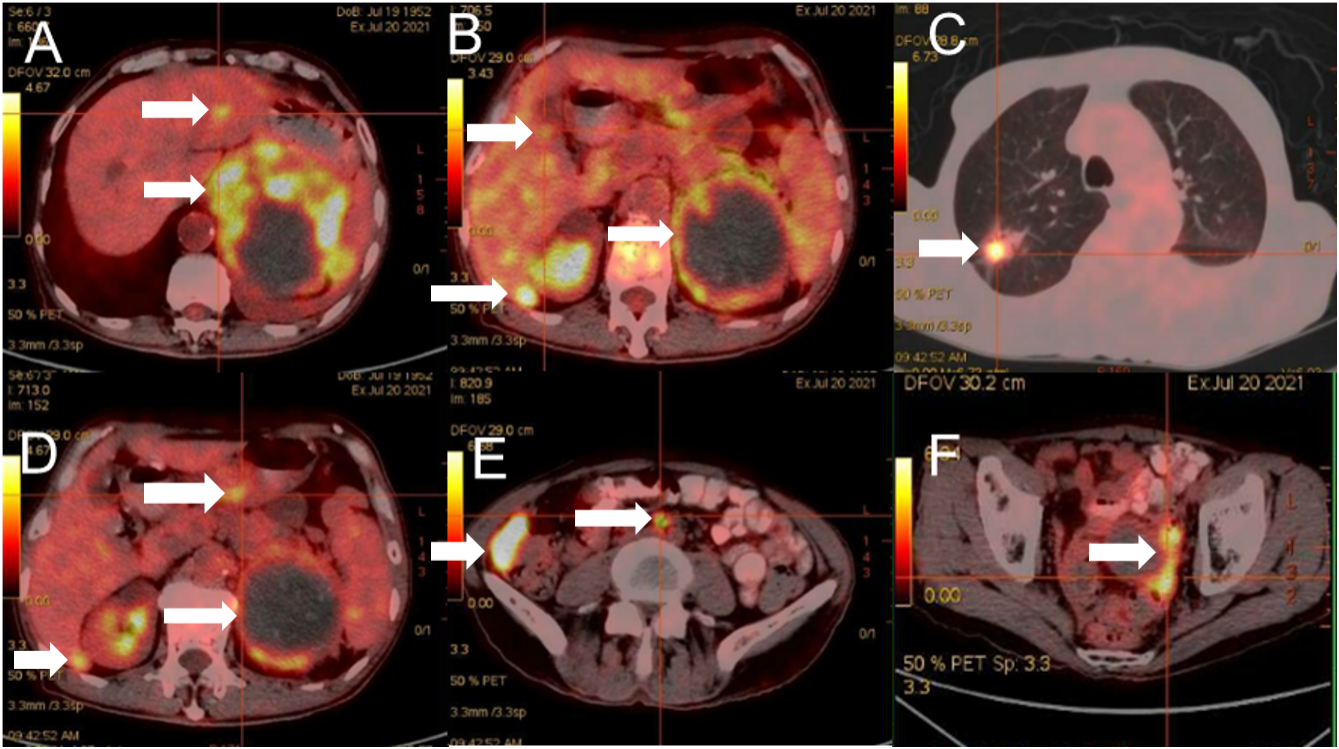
Positron emission tomography-CT showed a large, multi-loculated, hypermetabolic, cystic mass in the left adrenal region (A-F).
